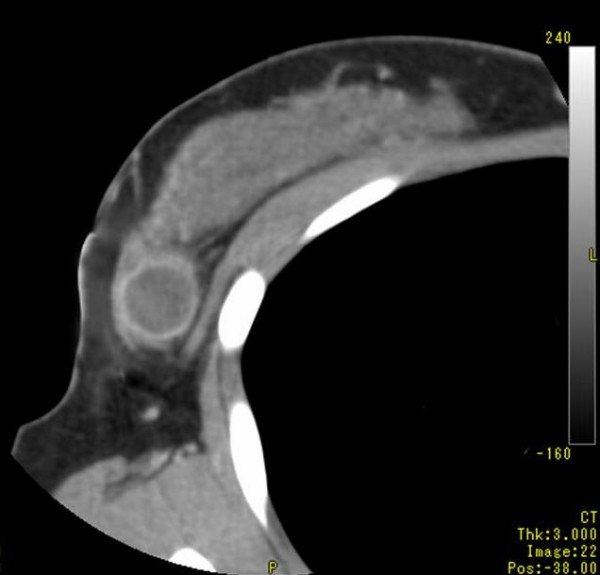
Computed tomography scan imaging (CT) of the tumor. Contrast-enhanced CT revealed, in the right lateral-upper quadrant, an irregularly shaped, 2,5 cm tumor showing peripheral ring-shaped contrast enhancement.
